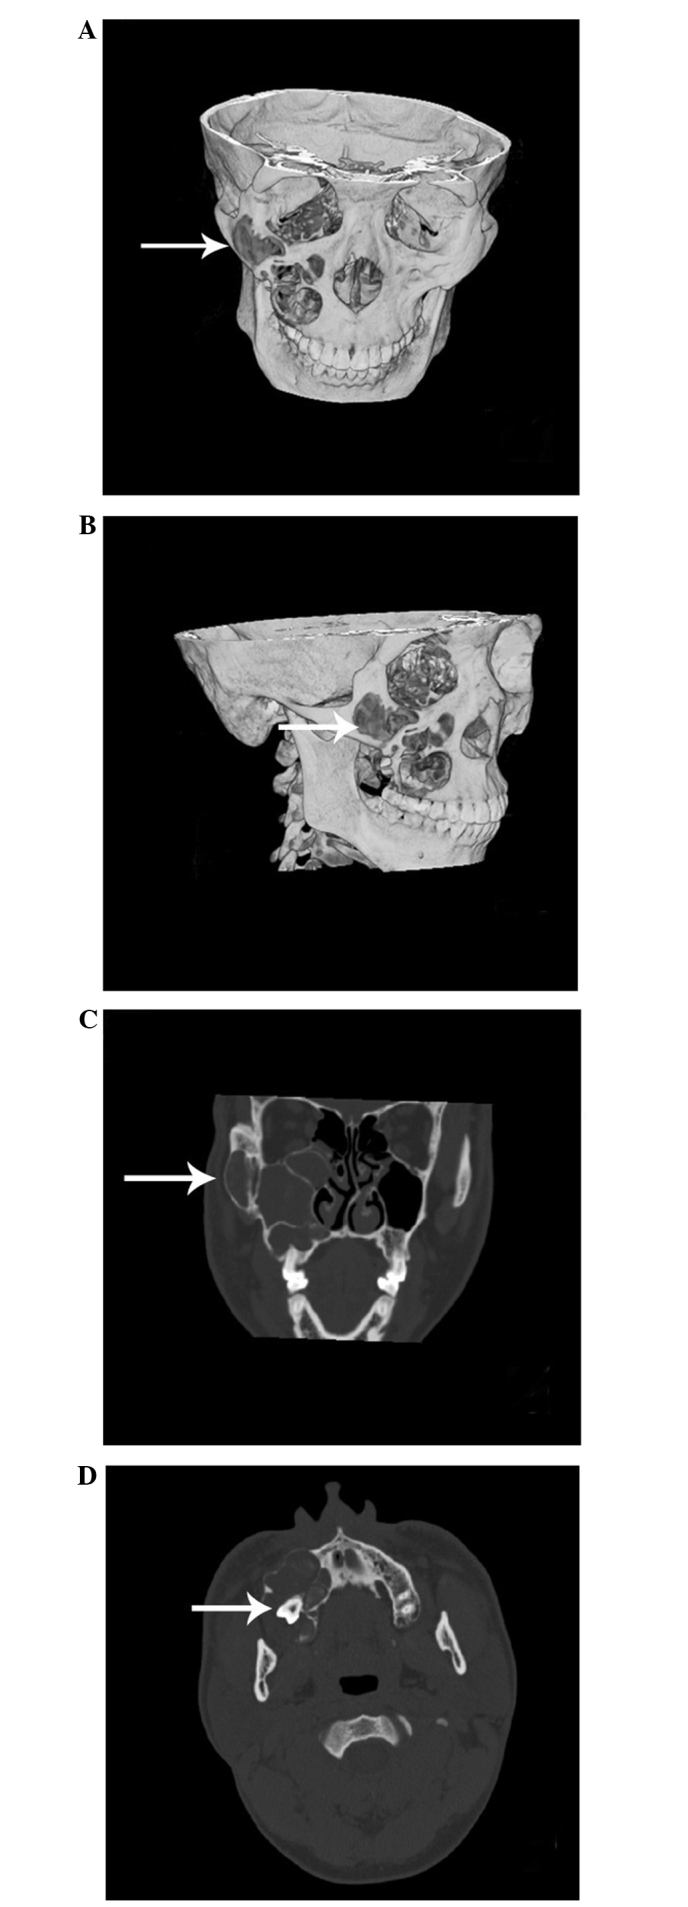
CT images. (A–C) Three-dimensional CT revealing a low density lesion located in the maxillary bone, zygomatic bone and maxillary sinus. (D) An axial scan revealing an impacted tooth. CT, computed tomography.
